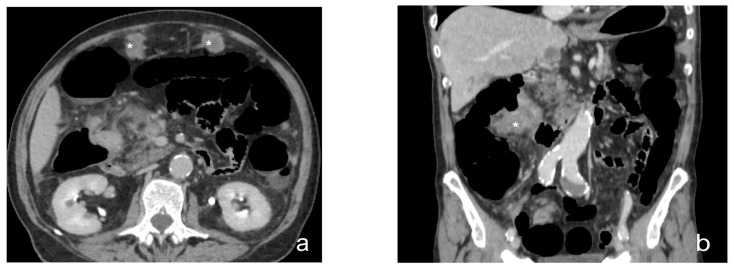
Contrast-enhanced CT scan, axial view (a), coronal view (b). Case of involvement of the root of the mesentery in a male, 63 y. Multiple and widespread peritoneal nodules (white star) present in all abdominal quadrants, with the largest in the mesogastric area.
